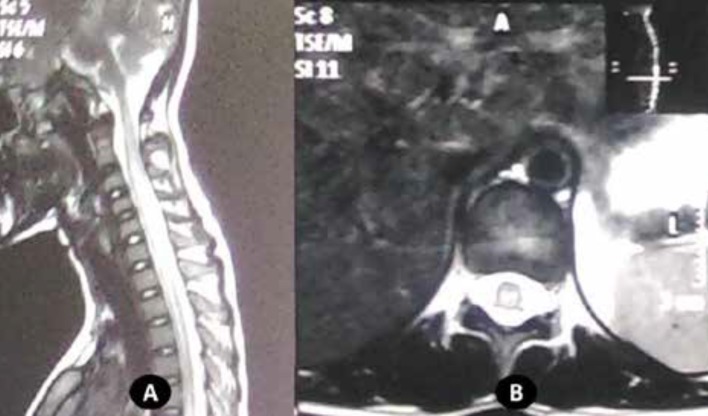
Saggital section (A) and Axial section (B) of MRI spine revealing extensive cord T2 hyperintensity extending from C2 to T1 suggestive of longitudinally extensive transverse myelitis (LETM)
